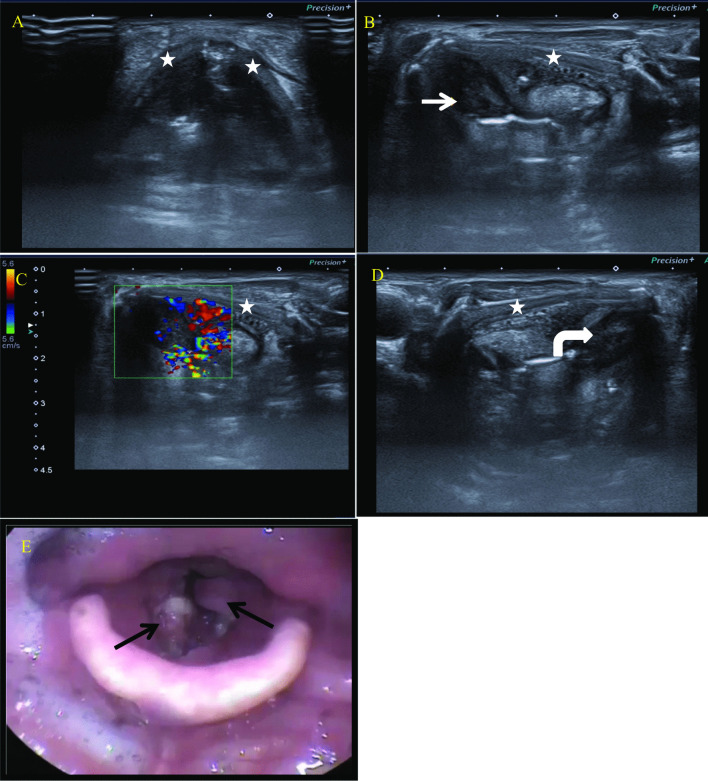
A 68-year-old heavy smoker, male patient presented with hoarseness of voice and stridor. A Laryngeal ultrasound anterior axial view shows the anatomical landmarks along the level of the true vocal cord with thyroid cartilage (marked by a star). B Laryngeal US, left lateral axial view, shows left glottic and supraglottic mass lesion (arrow) with ill-definition of the posterior aspect of the left thyroid lamina (marked by a star) near the midline, denoting erosion/invasion. C Colored Doppler assessment shows internal hypervascularity of the mass lesion. D Laryngeal US, right lateral axial view, shows right glottic and supraglottic mass lesion (curved arrow). E Flexible laryngoscopy of the same patient shows bilateral glottic and supraglottic mass lesions (black arrows). The anterior is at the bottom of this figure
